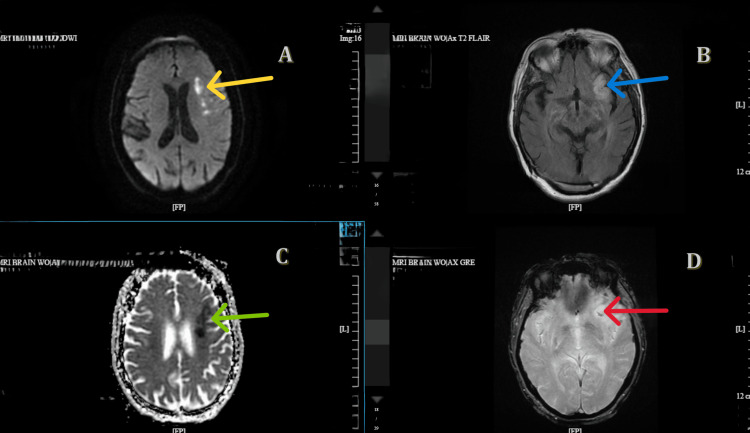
Findings concerning for acute ischemic infarct/stroke to early subacute ischemic infarct/stroke in left frontal lobe, with hemorrhagic transformation MRI of the brain showing acute ischemic infarct hyperintensity on DWI (yellow arrow in panel A), FLAIR (blue arrow in panel B), hypointensity on ADC (green arrow in panel C), and hemorrhagic changes (red arrow in panel D). DWI: diffusion-weighted imaging; FLAIR: fluid-attenuated inversion recovery; ADC: apparent diffusion coefficient
